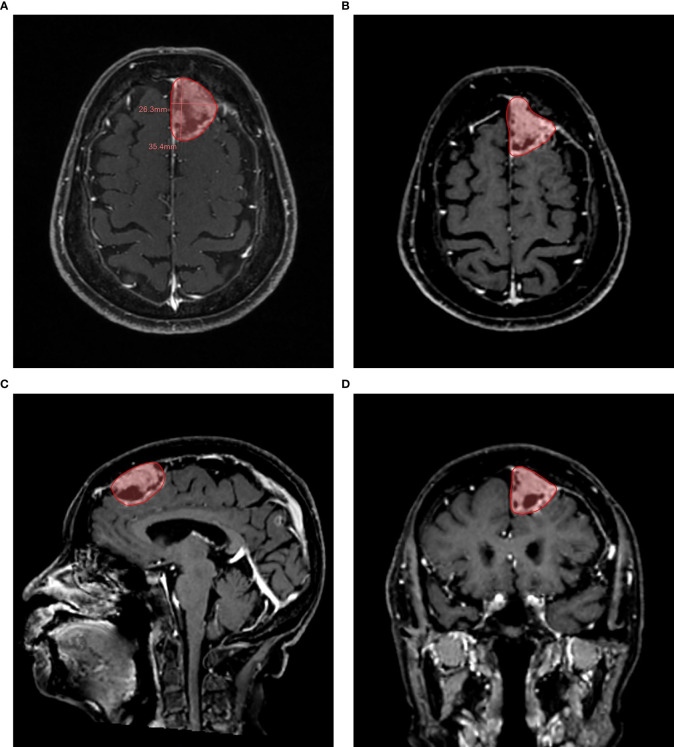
Example of a semi-automatic segmentation. The follow-up 3D CE-MRI of an 84-year-old female patient with brain metastases from lung adenocarcinoma. (A): Automatic measurement of maximum long diameter and maximum axial diameter. (B): Semi-automatic segmentation in axial plane. (C): Semi-automatic segmentation in sagittal plane. (D): Semi-automatic segmentation in coronal plane.
